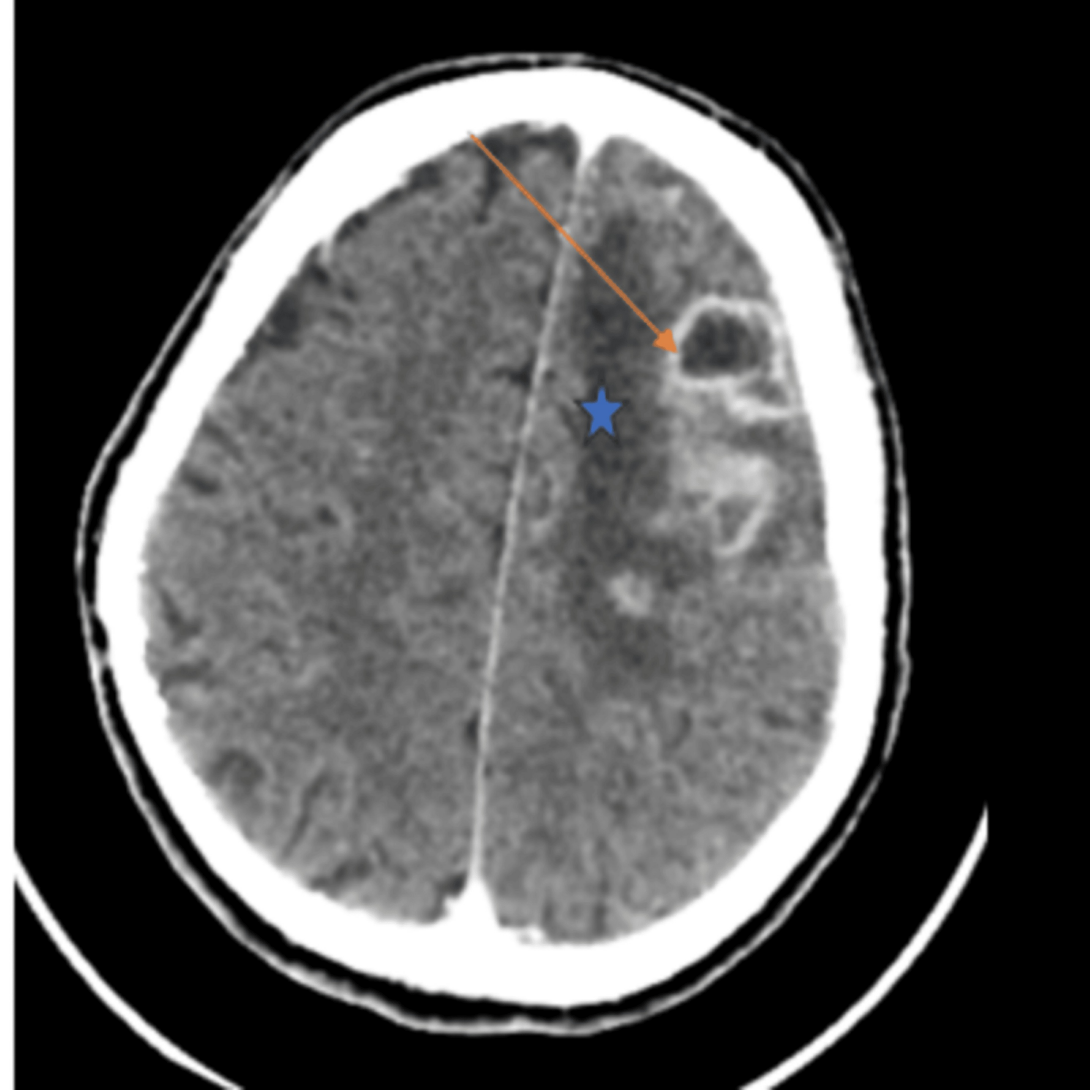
Cerebral CT images after injection of contrast showing left fronto-cortical and subcortical lesion (orange arrow) and peri-lesional edema (star)
